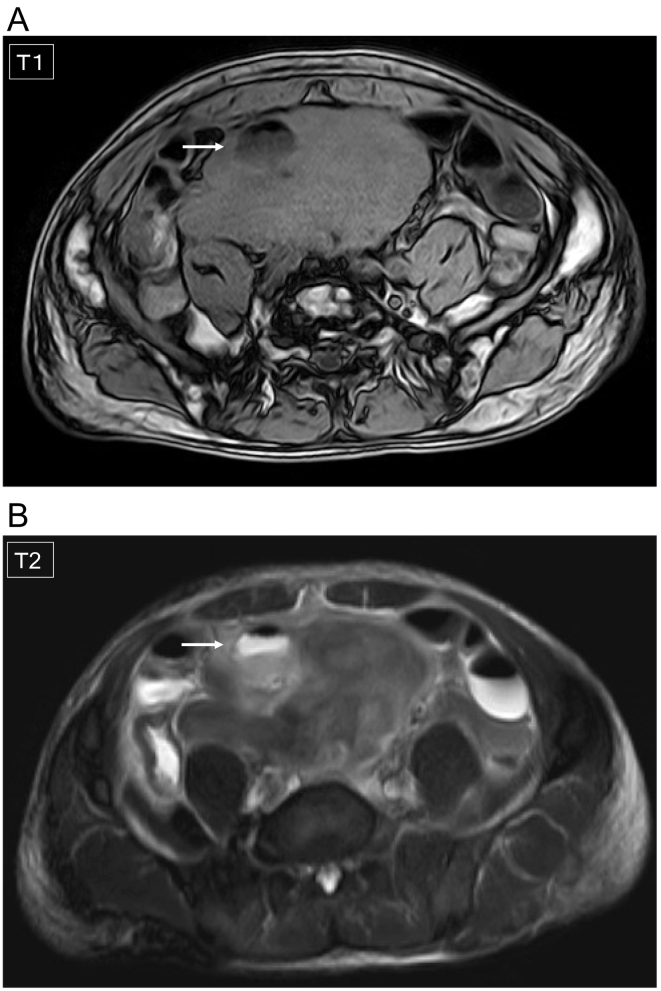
MRI examination revealed that the tumor had an internally non-uniform low signal on both T1- (A) and T2- (B) enhanced images, and fluid component retention was observed in the ruptured abscess (arrows).
